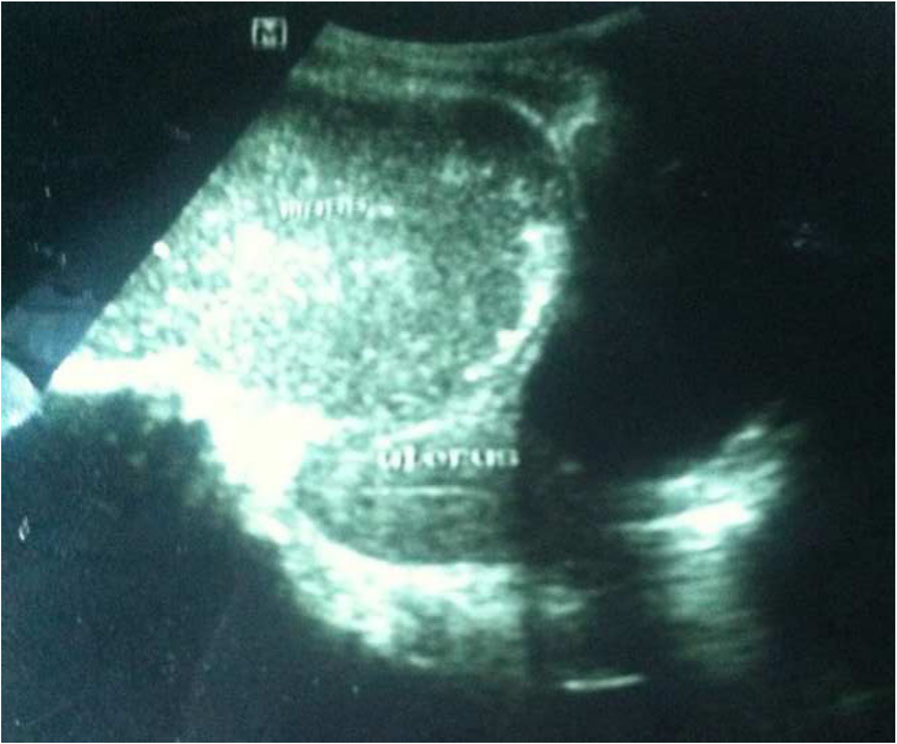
Ultrasound image of the hypo-echoic right adnexal mass
